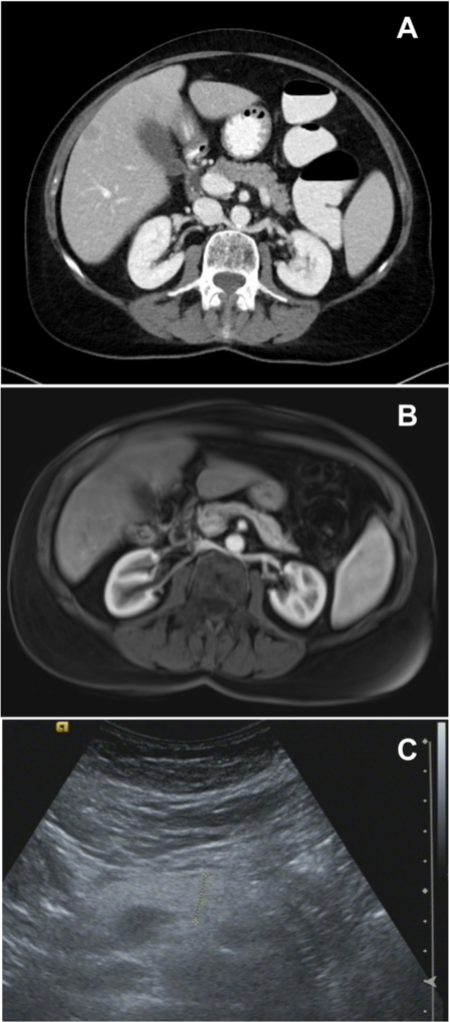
Abdominal imaging showing no evidence of pancreatic pathology. a CT. b MRI. c ultrasound
